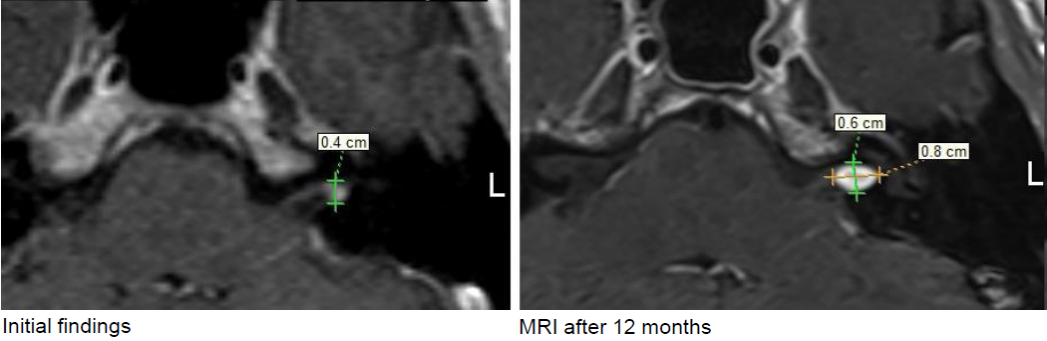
Intrameatal vestibular schwannoma, growing after 7 months from 4x4 mm to 8x6 mm; T1-w + CM, axial; A initial findings; B MRI control after 7 months.
